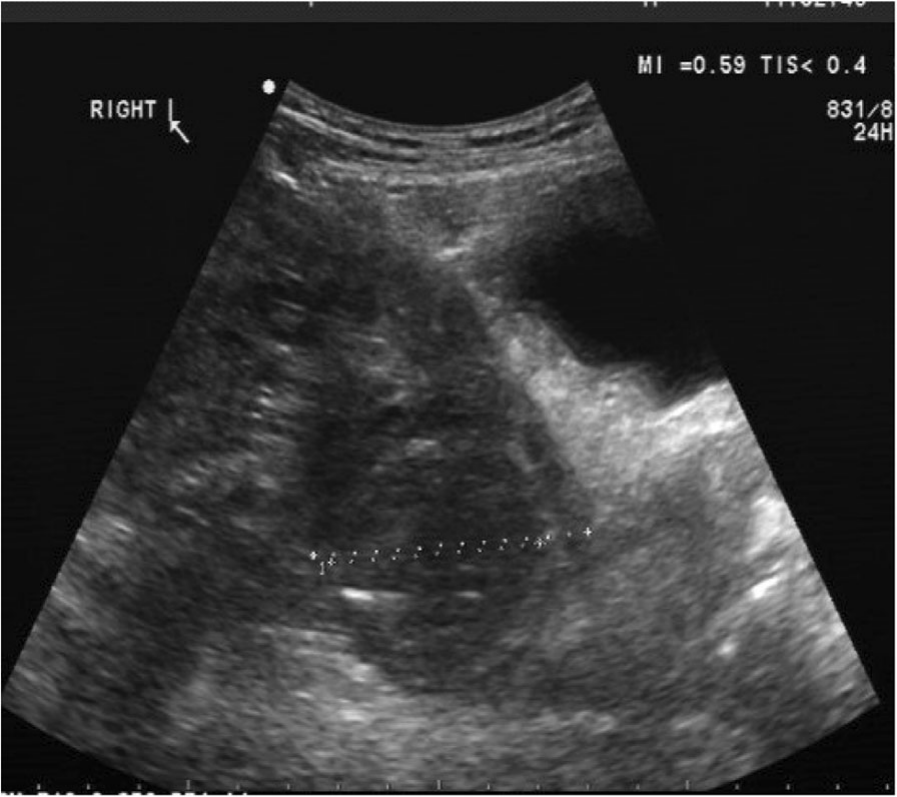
Transvaginal ultrasound image of the right uterine artery pseudoaneuyrsm
